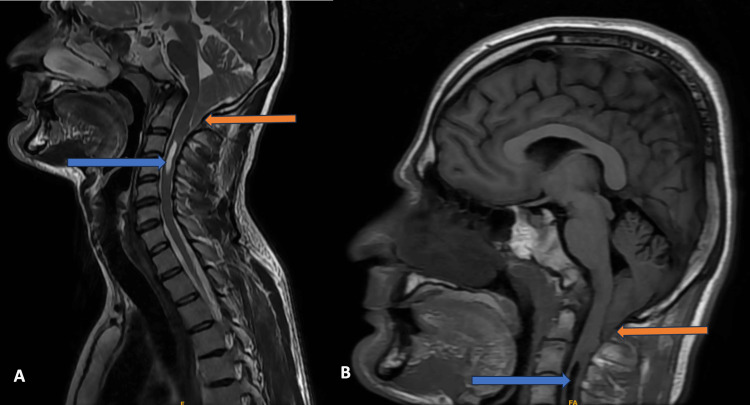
MRI of the cervical spine and brain Magnetic resonance imaging (MRI) of the cervical spine, mid-sagittal T2-weighted image, and MRI of the brain, mid-sagittal T1-weighted image, show tonsillar herniation (orange arrow) and hydrosyringomyelia (blue arrow), appearing hyperintense on T2 and hypointense on T1-weighted images (blue arrow) (A and B).
